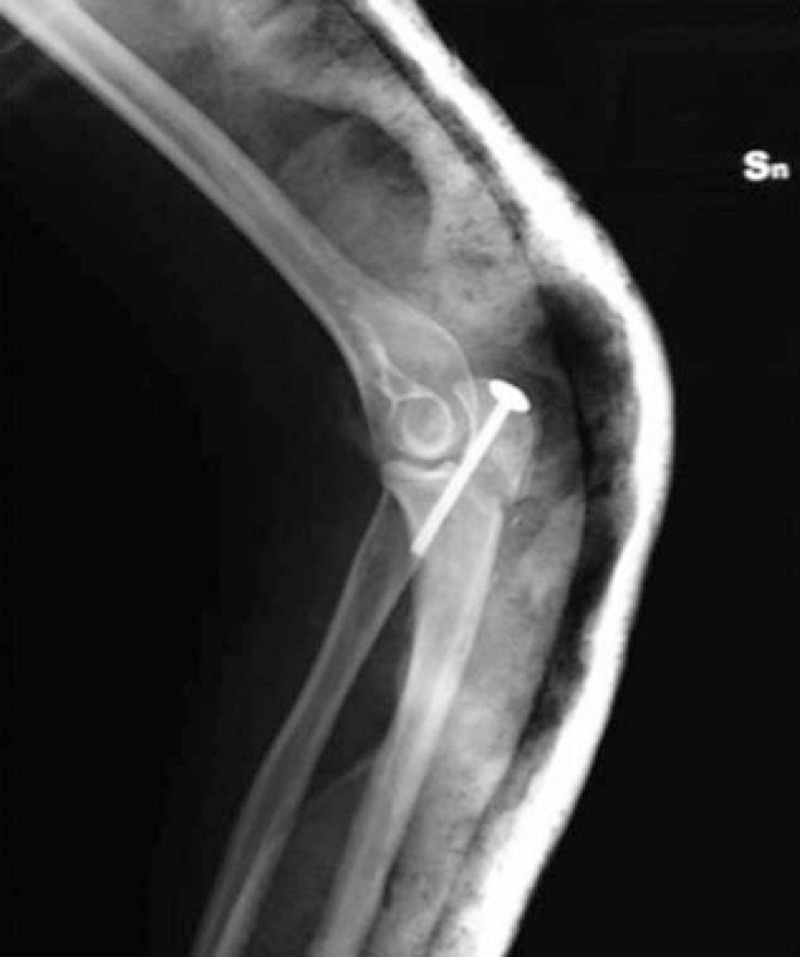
Postoperative X-ray shows the osteosynthesis performed using a single cannulated screw.
